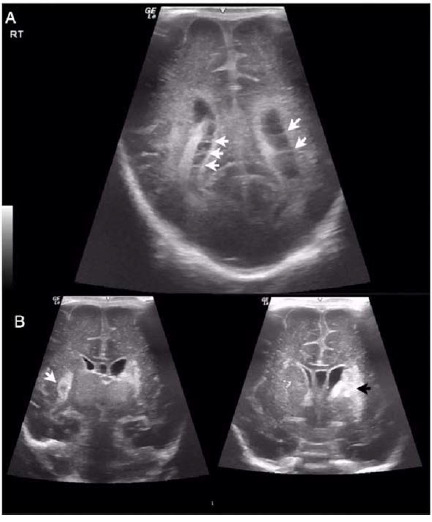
Ultrasonography images of the brain of triplet B following GBS sepsis at different time points. (A) Image taken on day 17 of life showing signs of ventriculitis characterized by thin septations (white arrowheads) and debris in the lateral ventricles, and turbidity in the cerebrospinal fluid. (B) Image taken at 1 month of age showing the development of bilateral parenchymal hemorrhages: a 2.3 × 2.0 cm hematoma in the left frontal cerebral region (black arrowhead) and a 1.8 × 1.3 cm hematoma in the right parietal periventricular area (white arrowhead).
